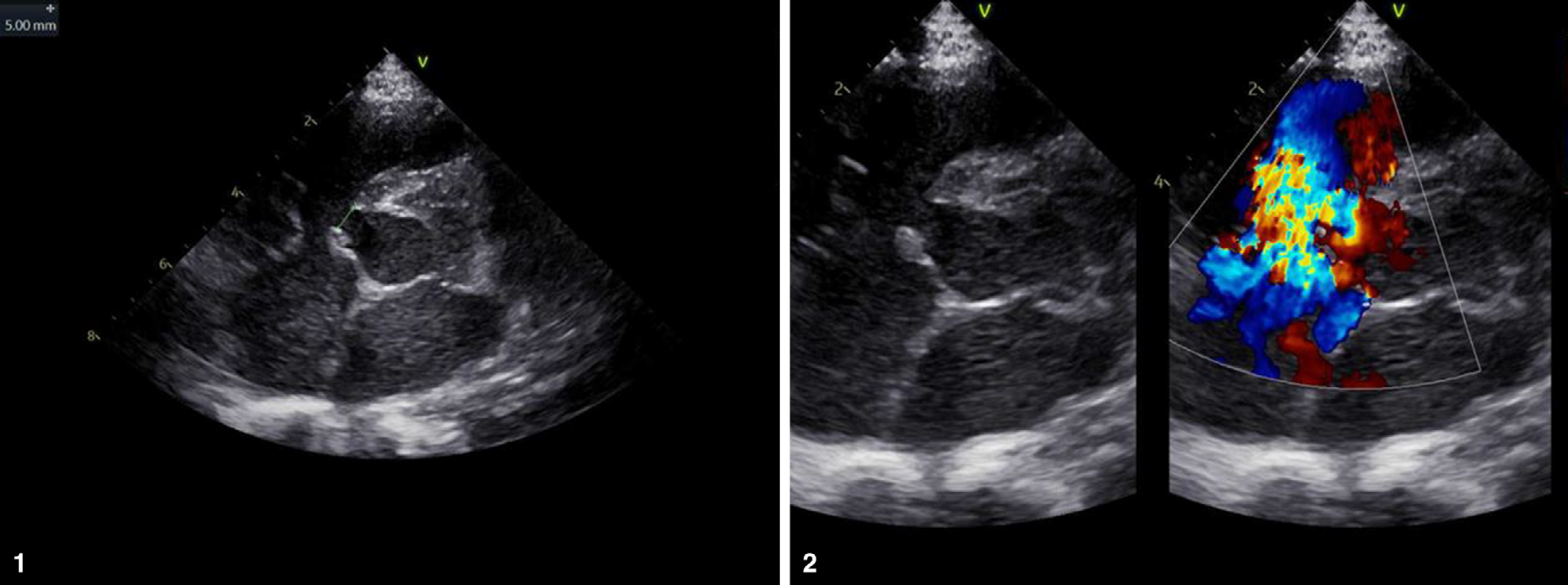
Preoperative transthoracic echocardiogram showing a perimembranous VSD with left‐to‐right shunt (arrow).
